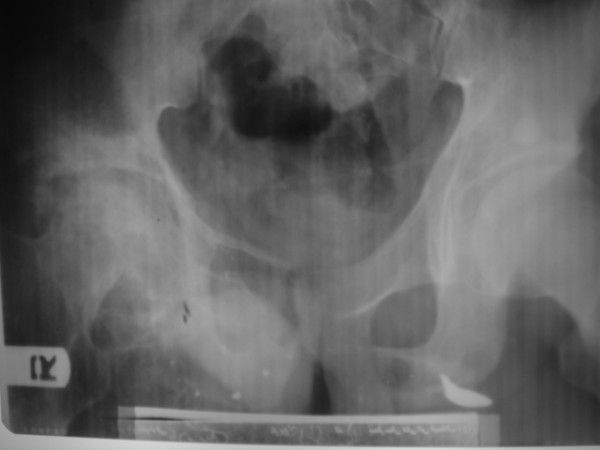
Plain X-ray of the right hip joint showing destruction of the joint cavity and femoral head.
